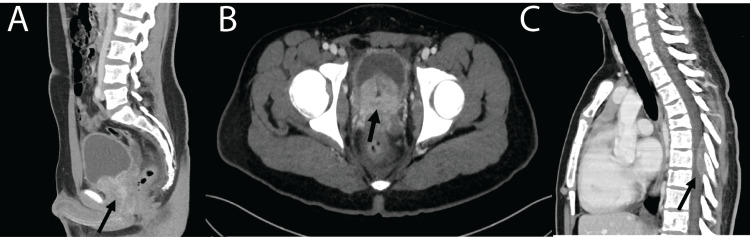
Initial non-contrast CT of the abdomen and pelvis A and B: Axial and sagittal non-contrast CT imaging demonstrating a large prostatic mass (black arrow) infiltrating the base of the bladder; C: Sagittal non-contrast CT of the chest demonstrating compression deformities of T4 and T5 along with intraspinal metastasis spanning from T6 to T8 (black arrow).
